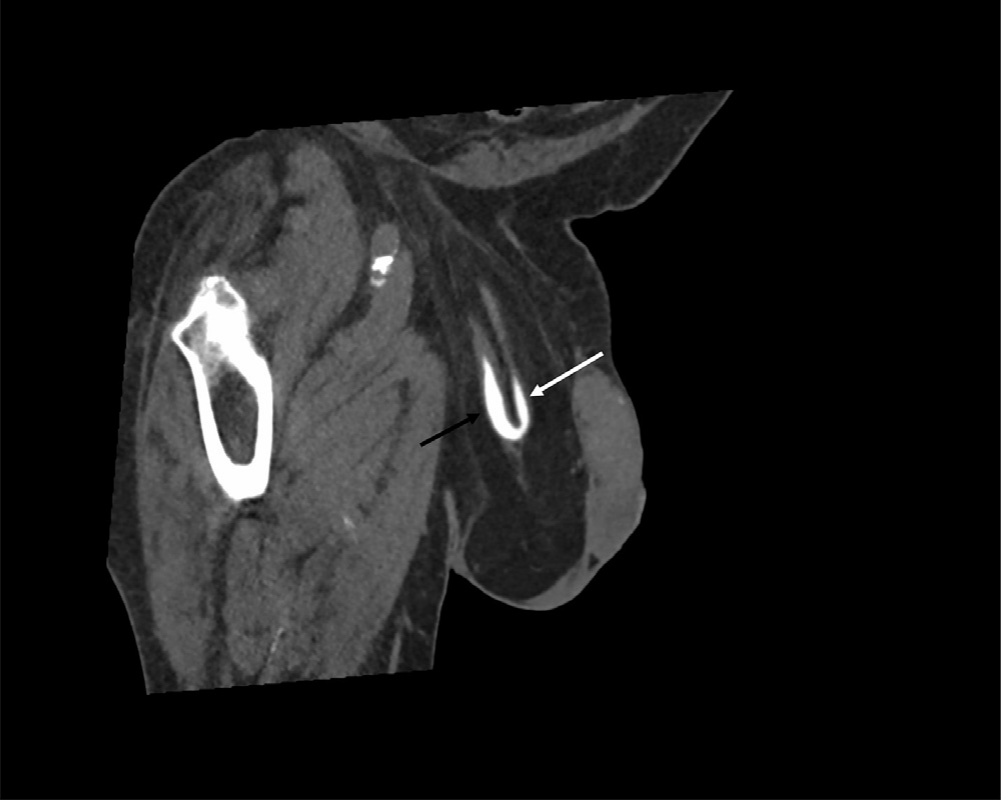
Delayed-phase CT with coronal oblique reformat. The inguinal hernia sac contains fat and the right ureter. The white arrow indicates the afferent loop, with the black arrow indicating the efferent loop.
